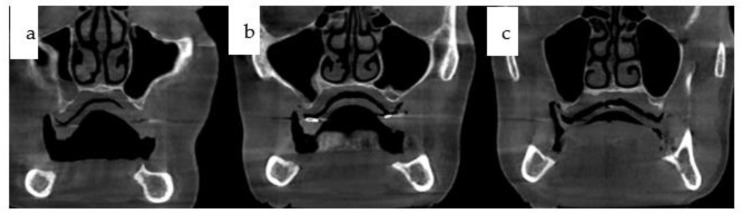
(a–c): Three cone-beam coronal views from the same patient in Figure 1 depict the reduced horizontal and vertical bone stock in the edentulous maxilla, displaying typical sequelae of atrophy-related centripetal bone loss.
